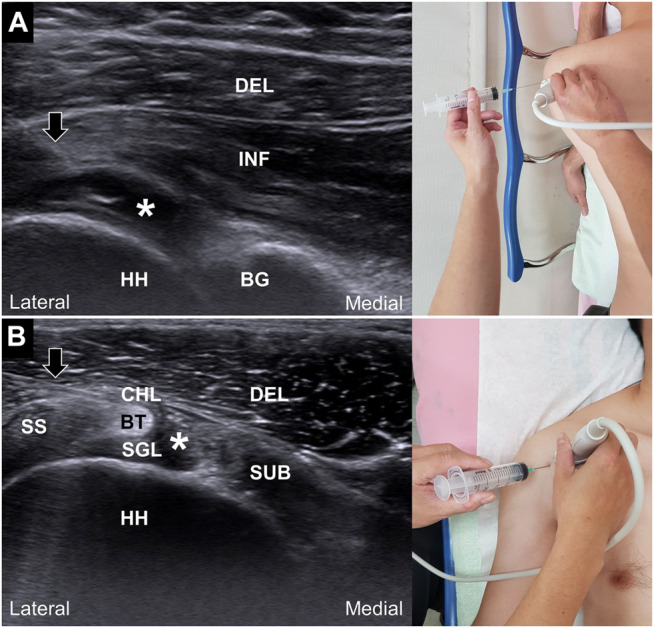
Ultrasound guided hydrodilatation through the posterior glenohumeral recess (A) and rotator cuff interval (B). Black arrows: needles; white asterisks: injectate; DEL: deltoid muscle; INF, infraspinatus muscle; HH, humeral head; BG, bony glenoid of the scapulae; SS, supraspinatus tendon; SUB, subscapularis tendon; CHL, coracohumeral ligament; BT, long head of the biceps tendon; SGL, superior glenohumeral ligament.
